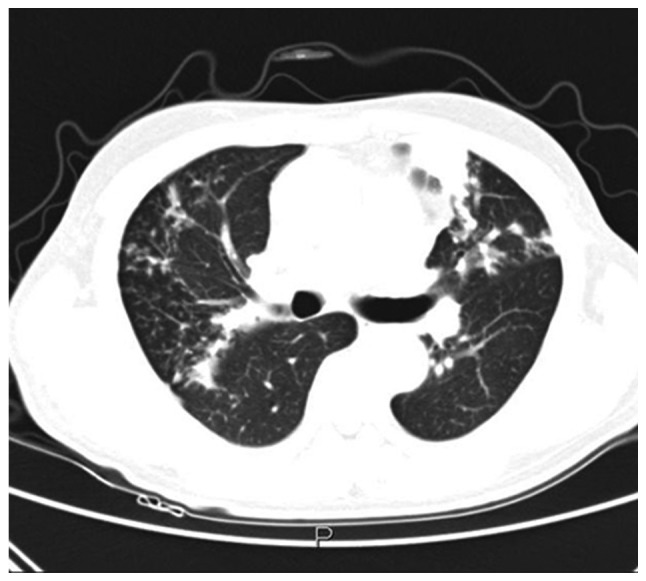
Initial Computed tomography (CT) scan of the lungs showing increased lung markings, plaques and nodules of varying sizes.
